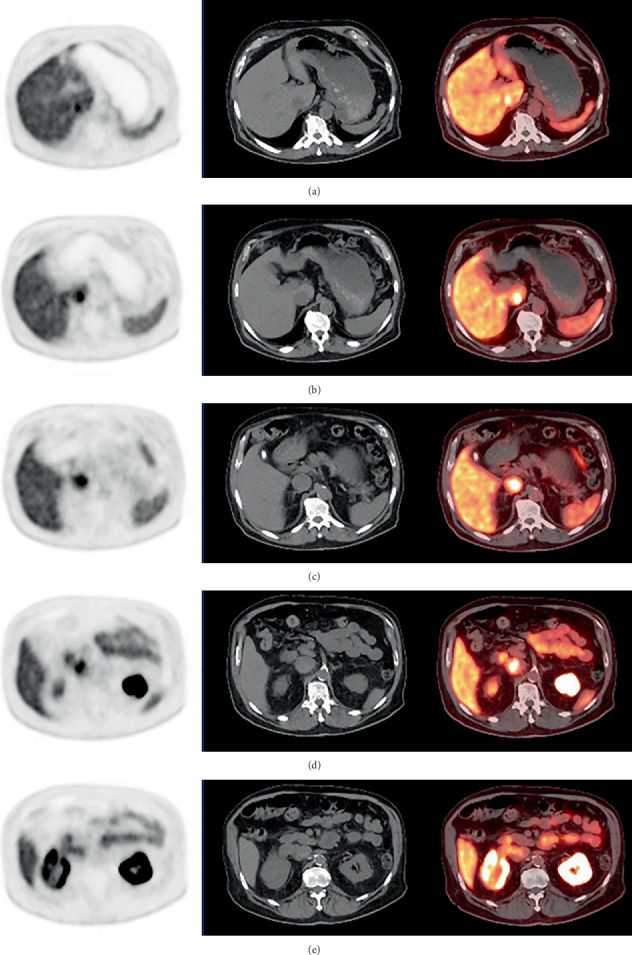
Axial PET, CT, and fused PET/CT images from the same baseline PSMA PET/CT (a–e) shown in Figure 1a with PSMA-avid tumor thrombus extending superiorly to the intrahepatic IVC (a) and inferiorly to the right renal vein (e). The suspected primary right renal mass appears relatively photopenic compared to the remainder of the kidney (e).
